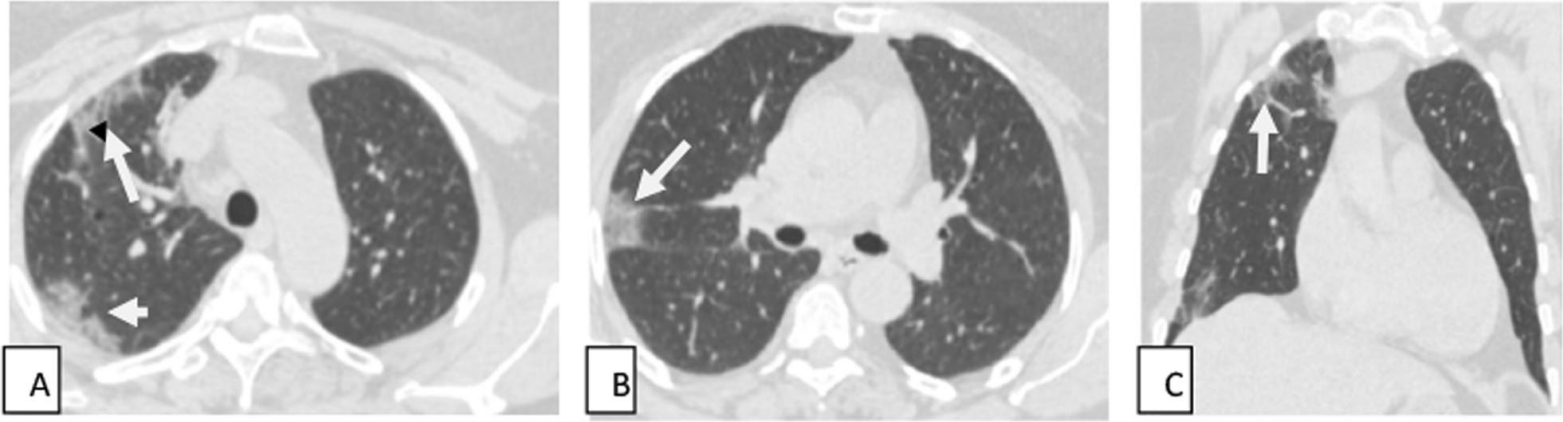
Male patient aged 56 years presented by loss of smell and taste with low-grade fever. Axial (A, B) and coronal (C) CT cuts show bilateral multifocal ground-glass opacities, and smaller areas of consolidation and areas of interstitial thickening giving crazy-paving appearance (white arrows) are seen involving both lung fields with peripheral/subpleural predominance (mild to moderate severity)
